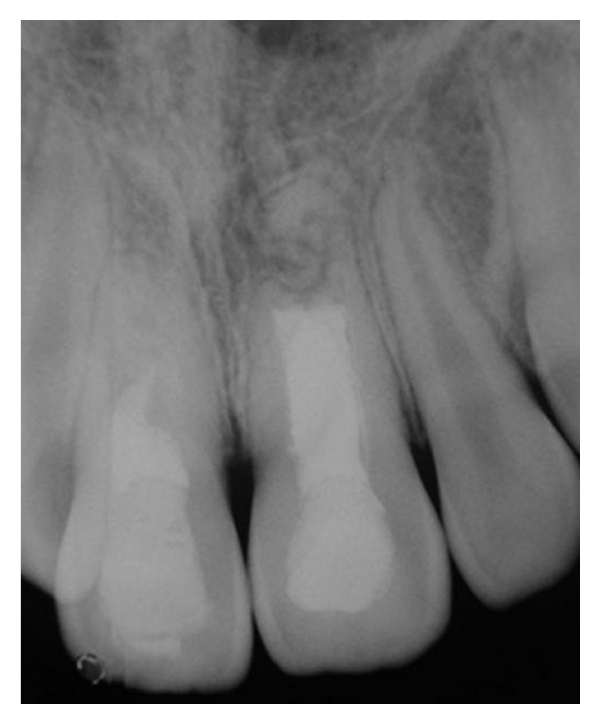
Three and half years posttreatment radiograph (June 2010).
